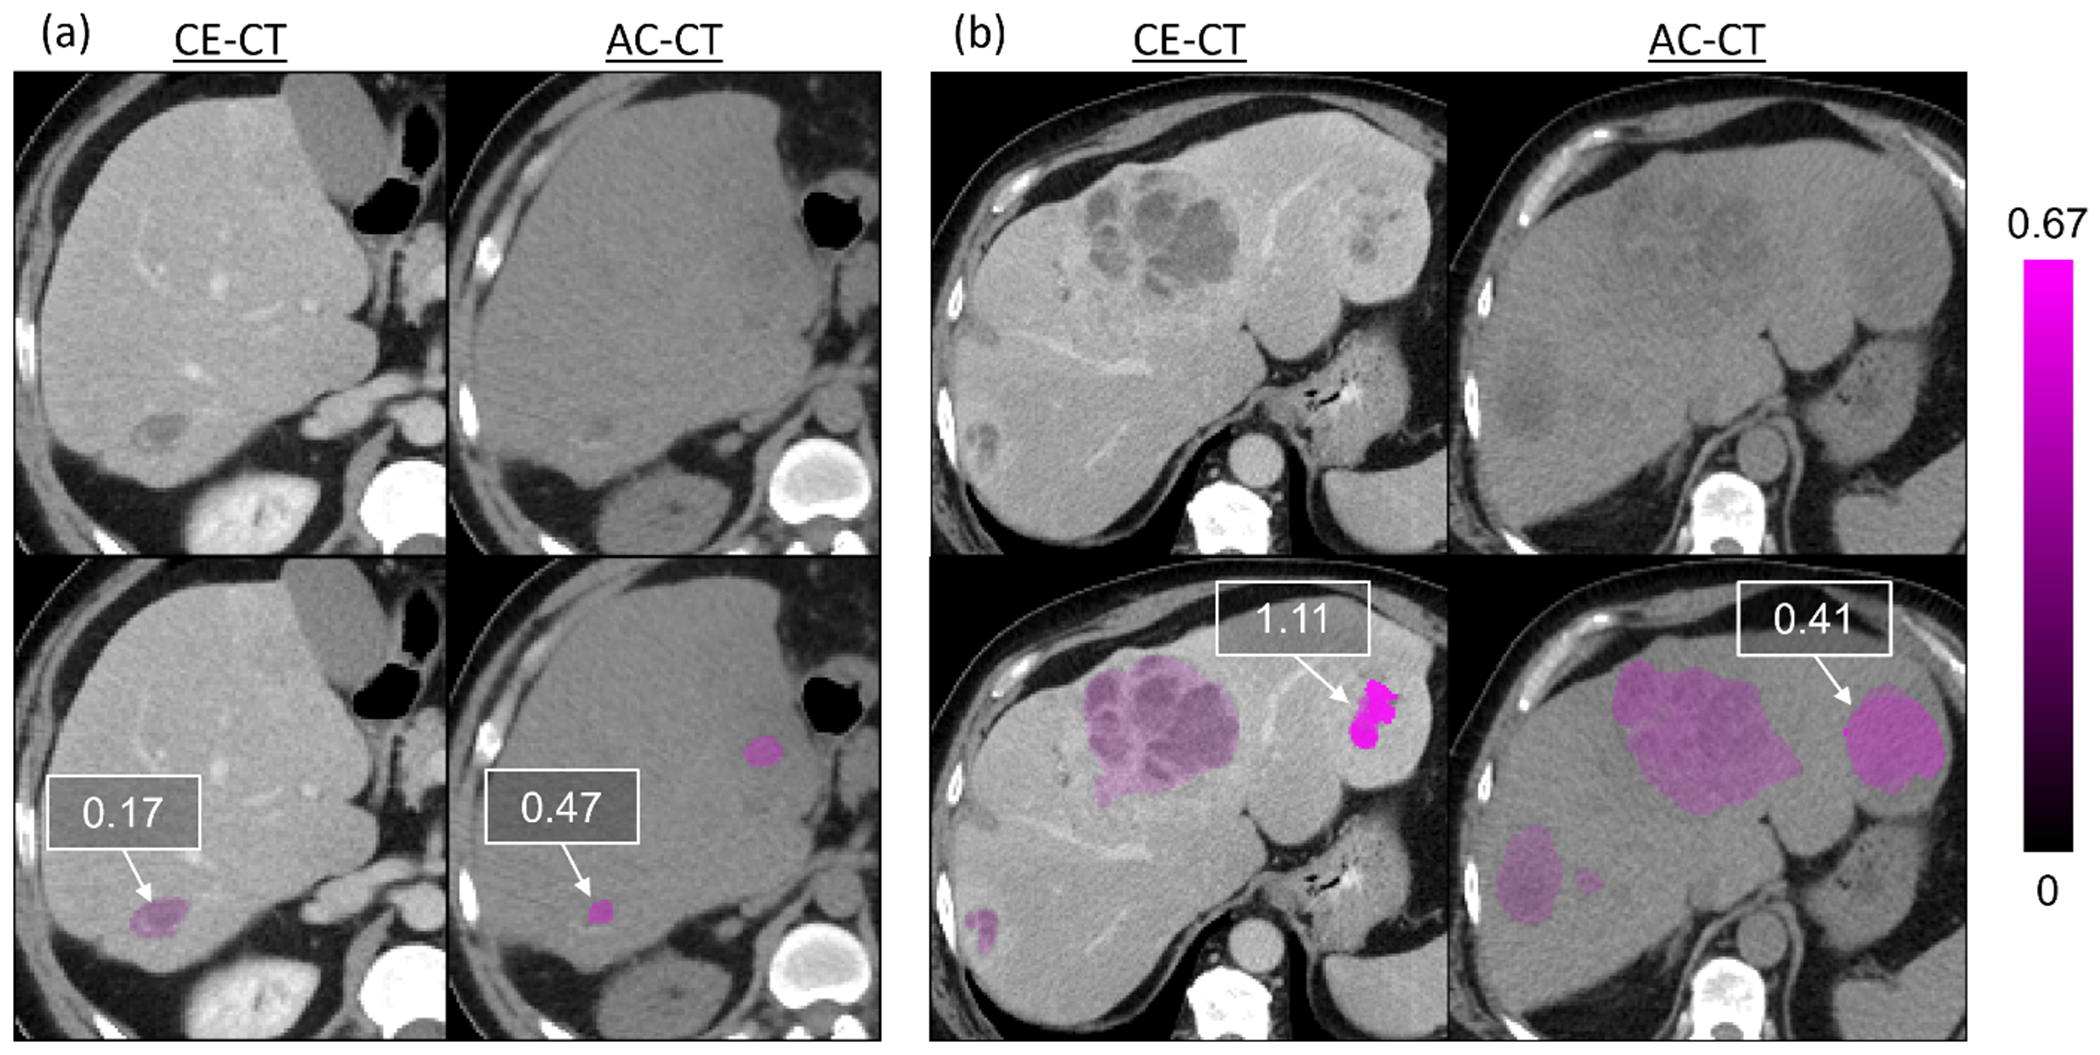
A qualitative evaluation of two example test scans with local gradients UQ measures (U_LG_) overlaid on predicted liver tumor regions. Figure (a) shows an example where the liver tumor is more visible in the CE-CT and has a lower uncertainty measure than the corresponding tumor and uncertainty measure on the AC-CT. Figure (b) shows an example where the uncertainty measure of a predicted tumor on the CE-CT has a higher uncertainty measure than the same tumor on the AC-CT. This discrepancy could be explained by the more heterogeneous presentation of the tumor on the CE-CT than on the AC-CT. U_LG_ uncertainty measure is listed in the box for each lesion. The color bar on the right indicates increasing U_LG_.
